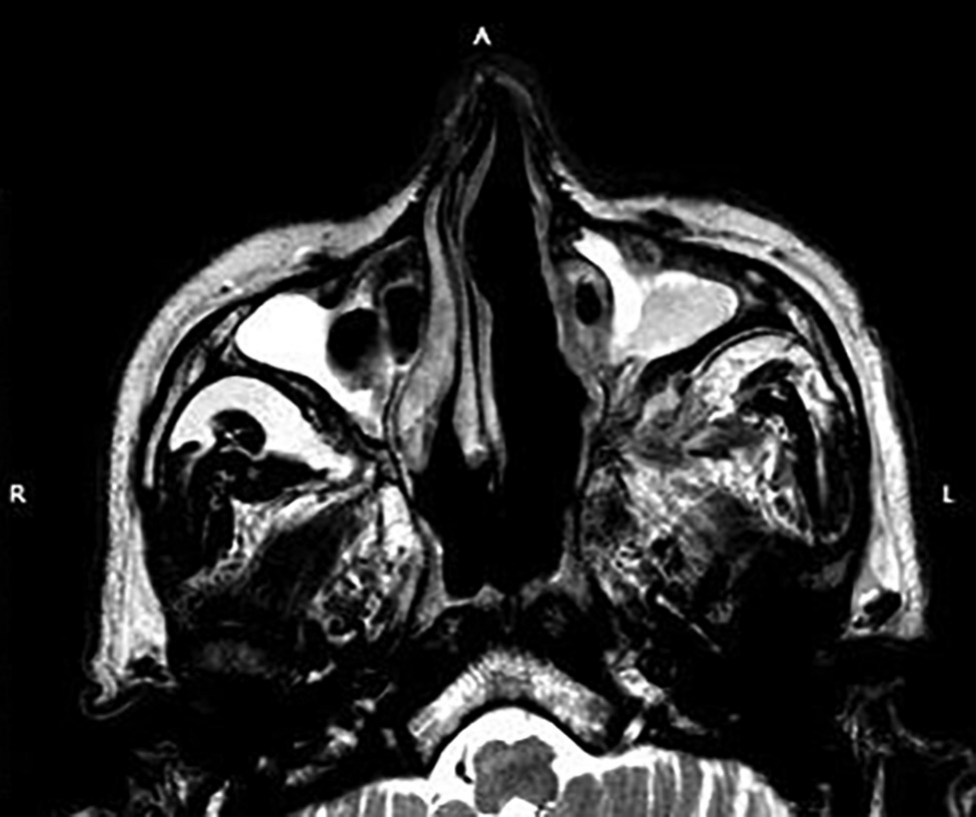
T2-weighted magnetic resonance imaging of the maxillary sinuses of a patient with mucormycosis after coronavirus disease, Pune, India, shows hypointense mucosal thickening bilaterally, more on the left side than the right. Near-complete occlusion of the sinus cavities and obliteration of left osteomeatal unit are seen. There is a mild deviation of the nasal septum with convexity toward the right side. There is mild soft tissue edema with altered signal abnormality involving the left pterygopalatine fossa extending to the left masticator space. L, left; R, right.
